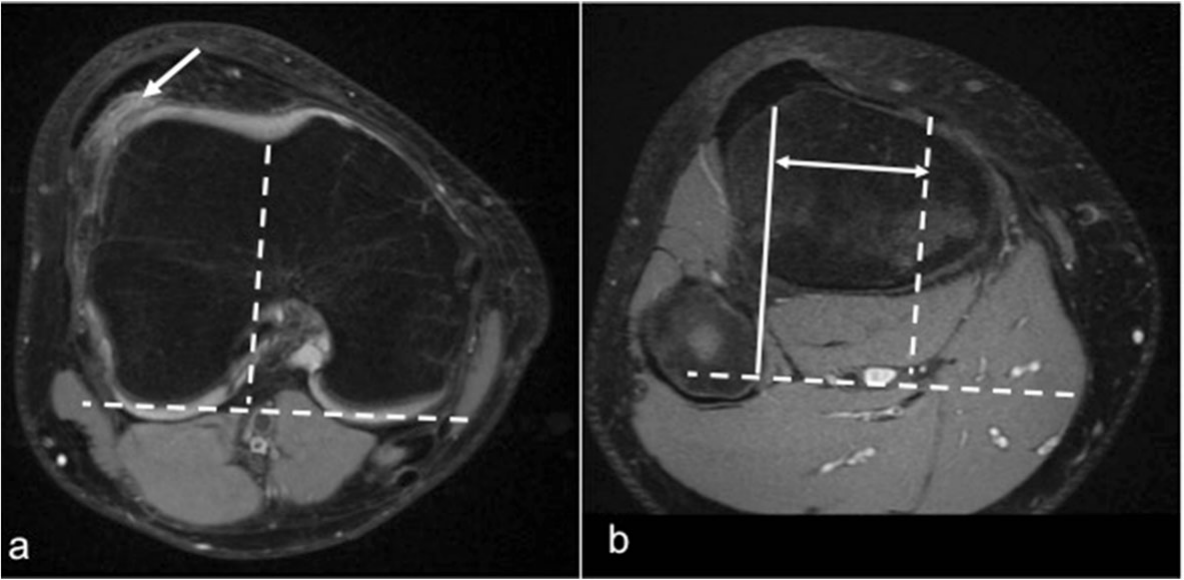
Tibial tubercle–trochlear groove distance (TT-TG) assessment. a Axial PDFS right knee MR image at the level of the trochlear groove. b Axial MRI at the level of the tibial tuberosity. TTTG is the distance between the solid and the dashed lines in (b). Note the edema in the superolateral aspect of Hoffa’s fat pad (arrow)
